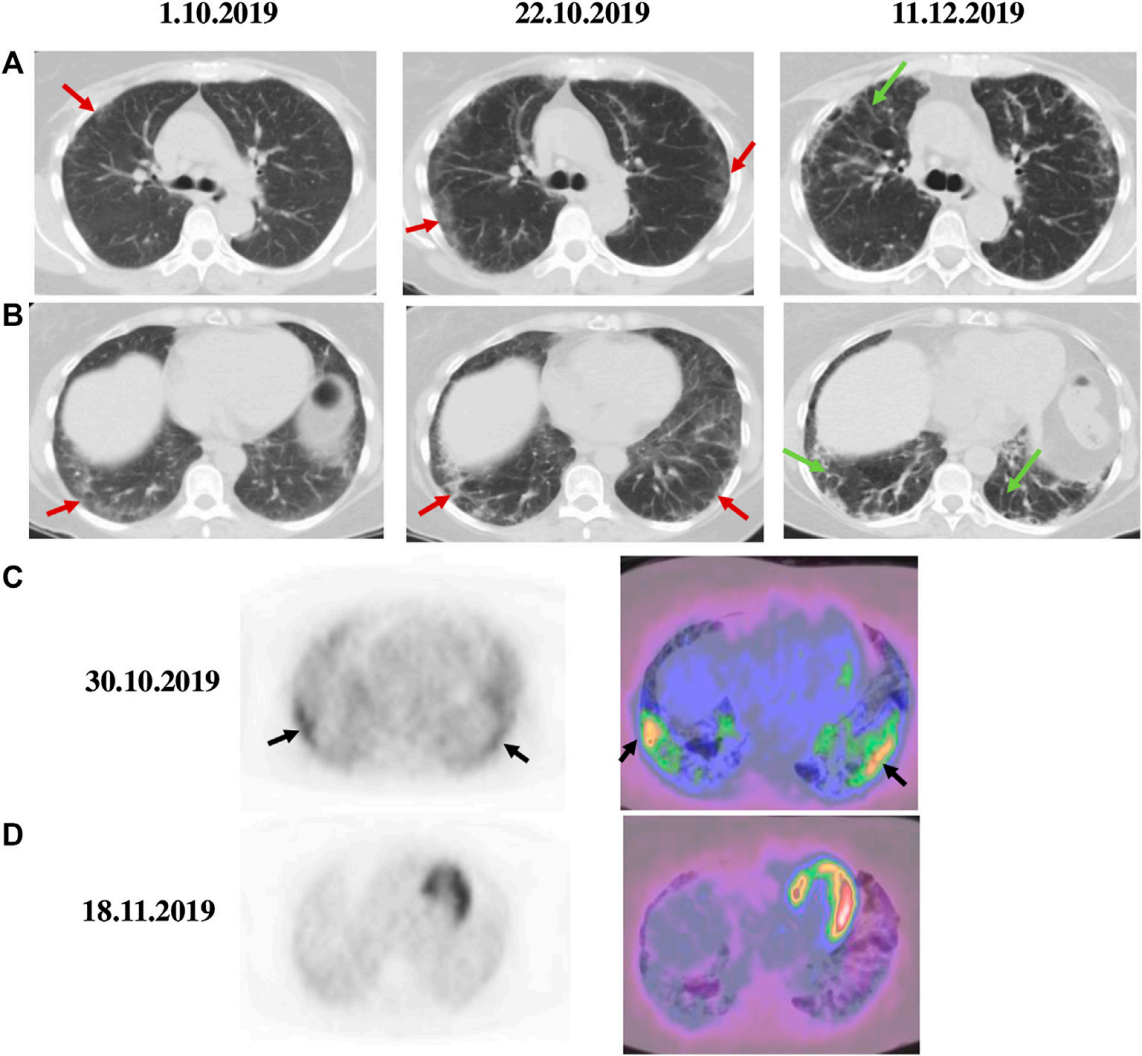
Chest CT and ^18^F-FDG PET/CT scans in anti-Jo-1 antibody-positive RPILD. Serial axial CT scans of upper and middle (A) as well as lower lobes of the lung (B) showed patchy ground–glass opacities (red arrows) on presentation (October 1, 2019) and worsening on hospital admission (October 22, 2019). Interlobular septal thickening (green arrows) was observed ∼6 weeks after starting therapy with tofacitinib (December 11, 2019). Axial PET (left) and fused PET/CT (right) images before (C) and after three weeks of therapy with tofacitinib (D) demonstrated a significant decrease in ^18^F-FDG uptake (SUV max from 4.2 to 1.9) as noted in the lower lung zones (black arrows). Tofacitinib was started on October 30, 2019 and nintedanib added on November 18, 2019.
